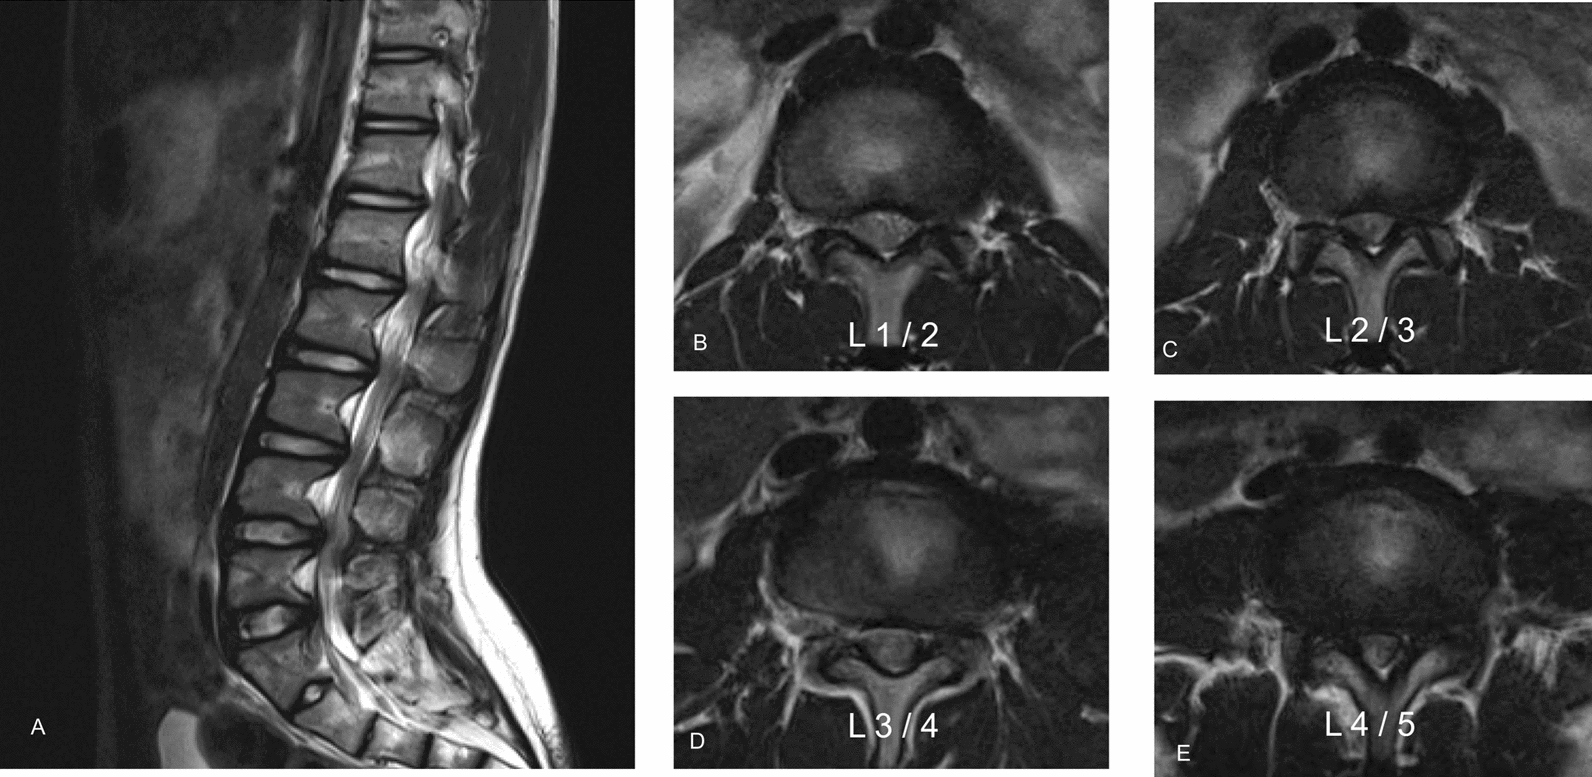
Preoperative magnetic resonance imaging of the thoracolumbar region revealed multilevel stenosis from T12 to S1, with a redundant nerve root sign (A: sagittal plane, B: transverse plane of L1/2, C: transverse plane of L2/3, D: transverse plane of L3/4, E: transverse plane of L4/5). The most stenosis was observed at the L4/5 level
